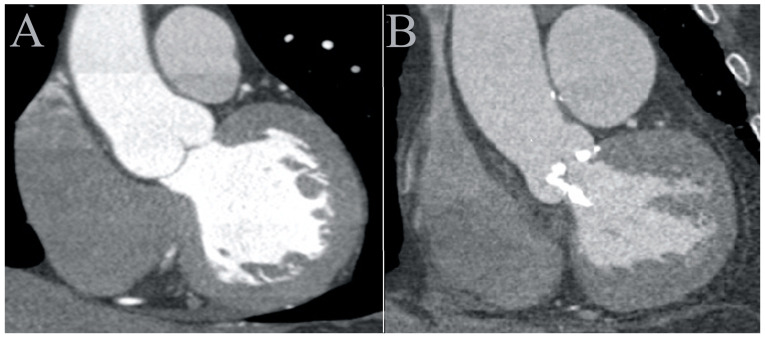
Frontal CCTA images (A) and (B) from a normal and stenotic aortic valve patient, respectively. Brighter (higher HU) contrast accumulation is evident in the normal valve patients in all regions. CCTA: cardiac computed tomography angiography; HU: Hounsfield unit.
